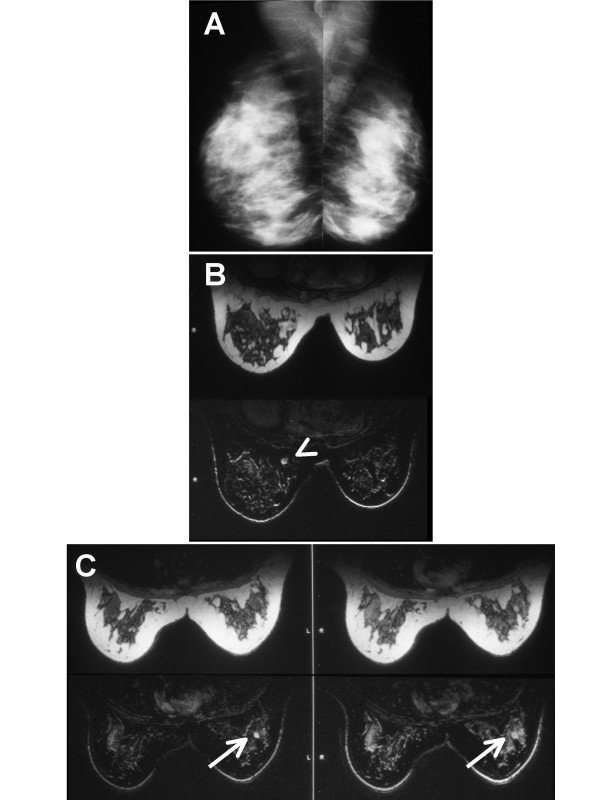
Imaging of subject 006. A) Subject 006 pre-MRI mammogram demonstrating heterogeneously dense breast tissue. There is no evidence of a cancerous lesion. B) Pre-contrast MR image showing an approximately 1.5 cm, smooth, round lesion in the right breast just above the nipple level medial and close to the chest wall (arrowhead). Core biopsy of this lesion demonstrated benign pathology, specifically, fibrosis with focal ductal epithelial hyperplasia [44]. C) Post-contrast MR images showing a small (approximately 1 cm), round, well-delineated enhancing mass (arrow) in the left breast at the 1:00 position. This mass was seen on both the initial delay after contrast injection (left) and the delayed contrast enhanced subtraction images (right). Core biopsy of this lesion indicated infiltrating ductal carcinoma, which was confirmed after removal via modified radical mastectomy [44].
